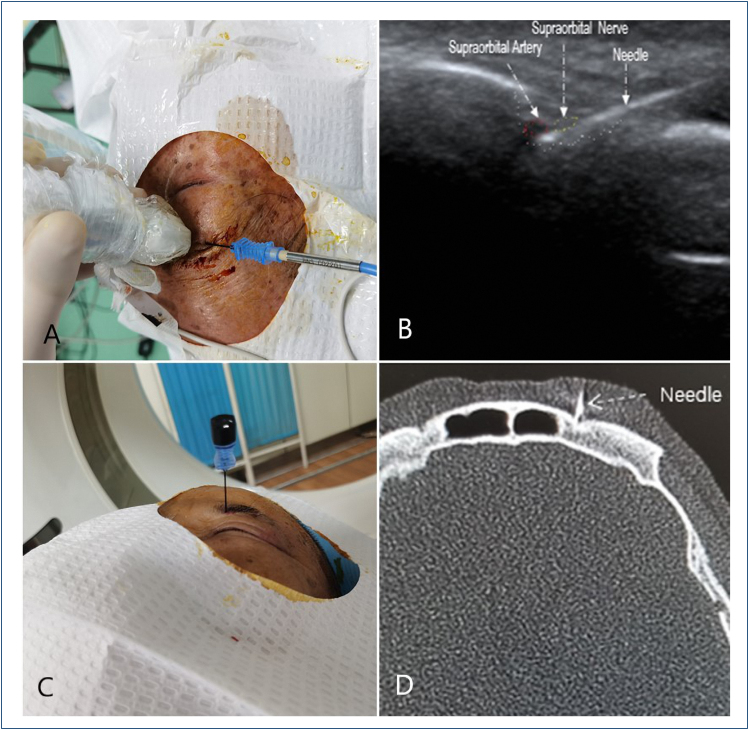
(A) Puncture operation of ultrasound-guided pulsed radiofrequency. (B) Ultrasound image of puncturing. (C) Puncture operation of computed tomography-guided pulsed radiofrequency. (D) Computed tomography image of puncturing.
